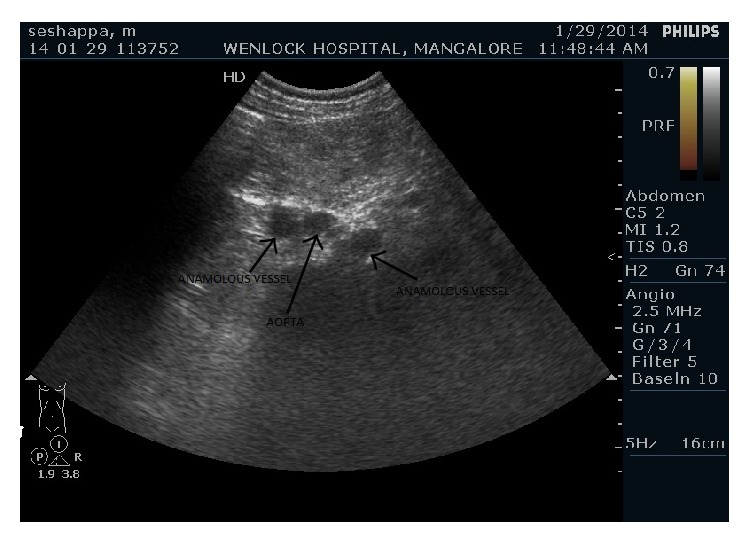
Grey scale ultrasound examination of abdomen shows evidence of anomalous vessel on either side of the lower abdominal aorta.
